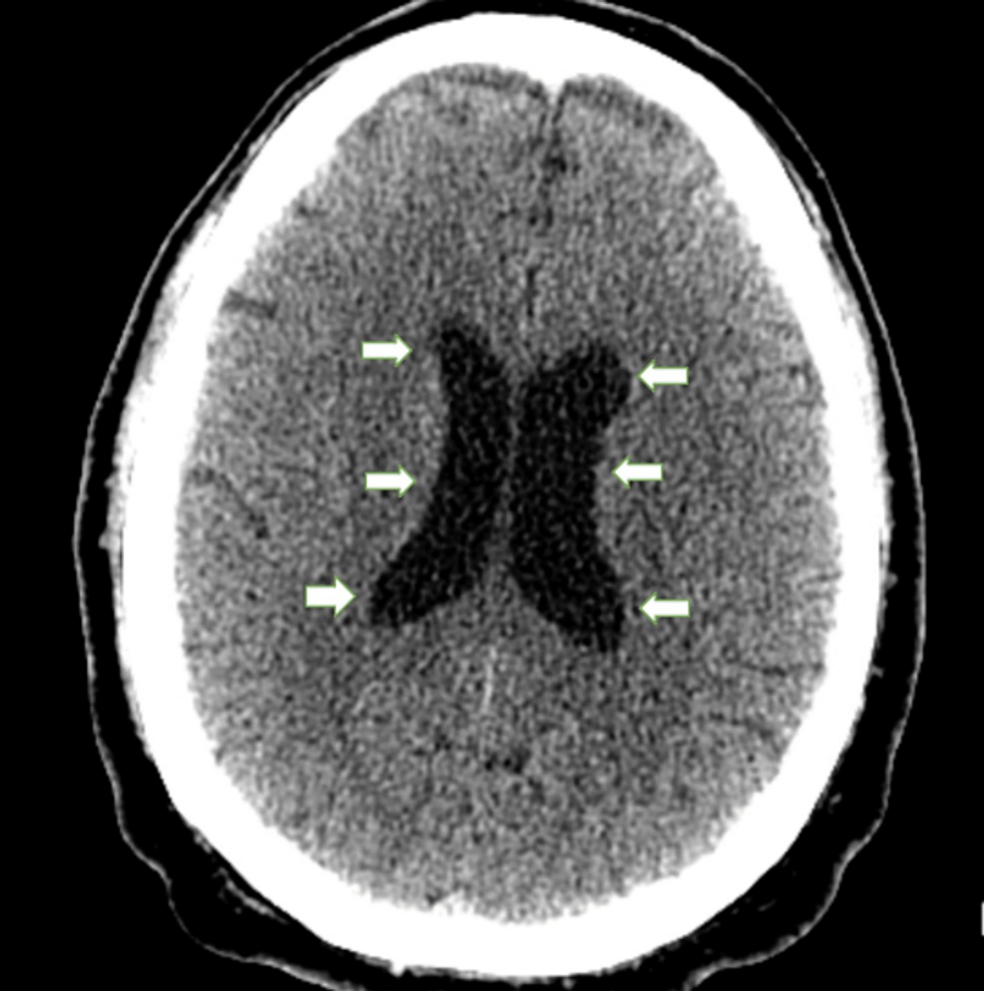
Head CT without contrast White arrows pointing towards dilated ventricles. No evidence of intracranial hemorrhage, midline shift, mass, infarcts, or acute changes were appreciated.
